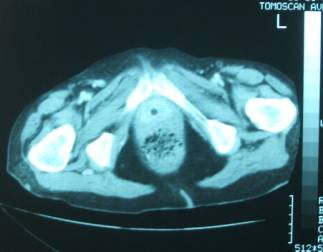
CT image showing thickened bladder wall with minimal lumen.
